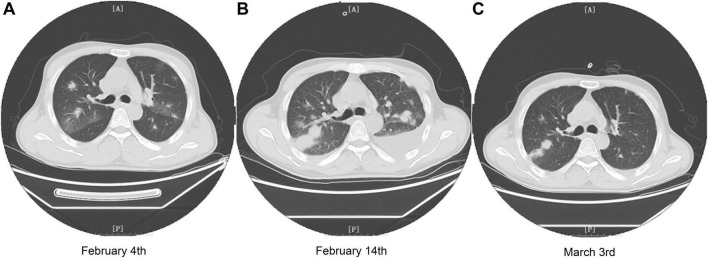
Chest CT images of the patient during treatment in our hospital. (A) Chest CT image of the patient on February 4th (2 days before VV116 initiation). (B) Chest CT image of the patient on February 14th, 4 days after completing the VV116 course. (C) Chest CT image of the patient on March 3rd, 3 days before leaving our hospital.
